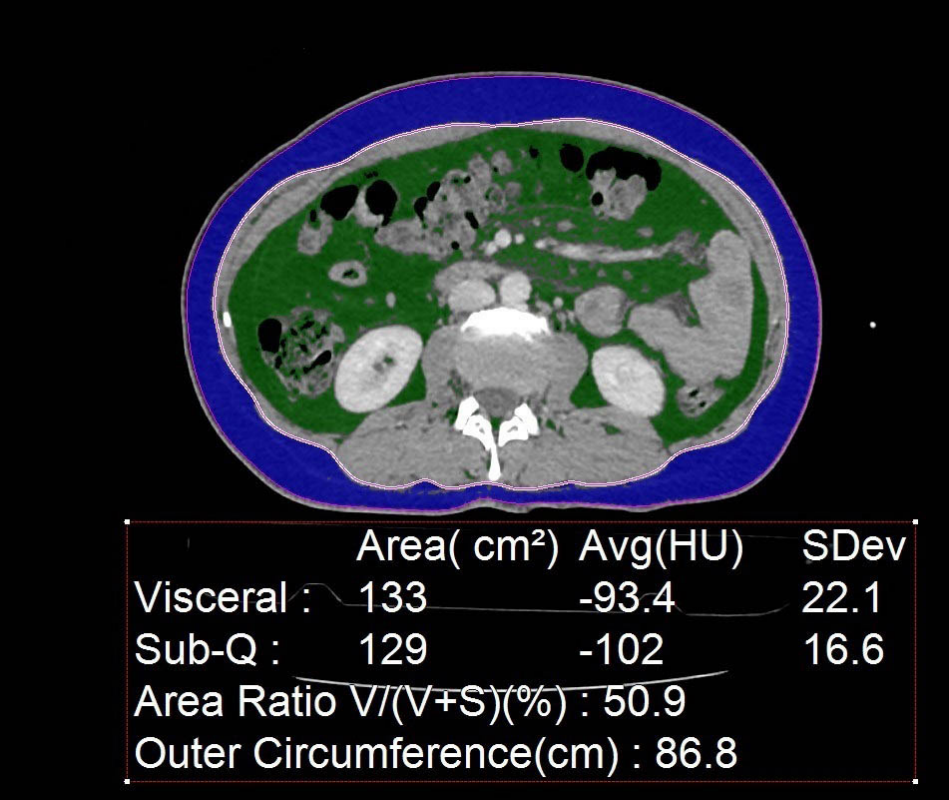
CT image at the L2 vertebral level shows segmentation
